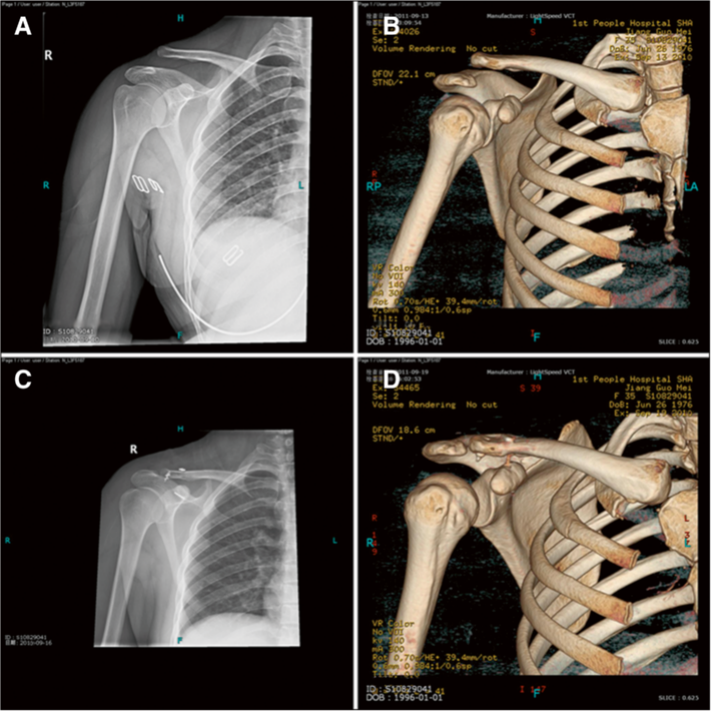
Treatment of Rockwood type V dislocation. (A,B) Preoperative X-ray and CT scan indicate Rockwood type V acromioclavicular joint dislocation. (C,D) Postoperative X-ray and CT scan reveal complete reduction of the dislocation.
